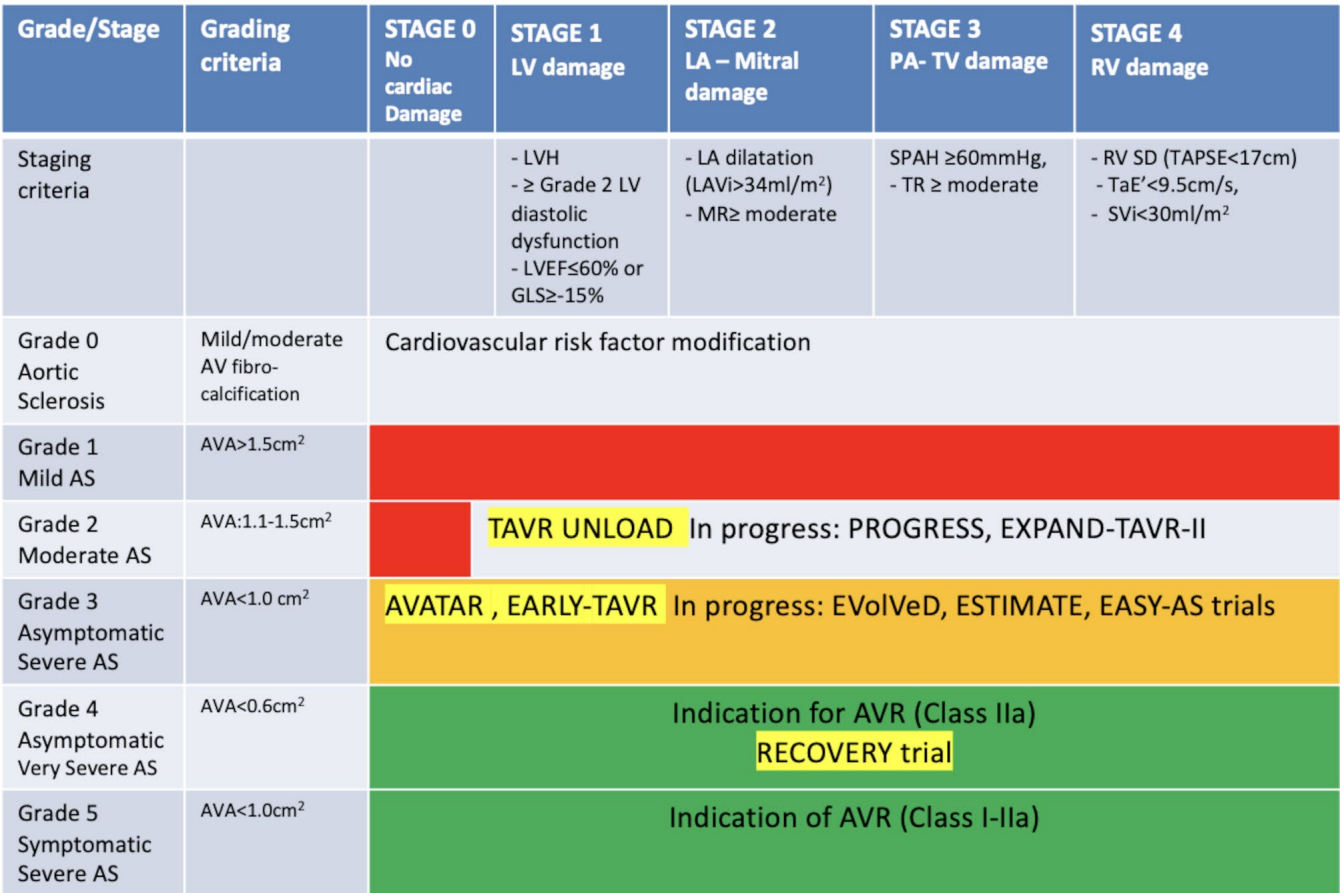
Proposal - Grading and Staging aortic valve stenosis in patients with preserved left ventricular ejection fraction PROGRESS- NCT04889872 at Clinical Trials.gov, TAVR UNLOAD: NCT02661451, EXPAND-TAVR-II: NCT05149755, AVATAR: NCT02436655, EARLY-TAVR: NCT03042104, EVolVeD: NCT03094143, ESTIMATE: NCT02627391, EASY-AS: NCT04204915, RECOVERY: NCT01161732 AVR: aortic valve replacement AVA: aortic valve area, LVH: left ventricular hypertrophy, LVEF: left ventricular ejection fraction, GLS: LV global longitudinal strain, LA: left atrium, LAVi: left atrial volume index, MR: mitral regurgitation, SPAH: systolic pulmonary pressure, TR: tricuspid regurgitation, RV: right ventricle, SD: systolic dysfunction, TaE’: Tricuspid annular E’ Doppler velocity, SVi: Stroke volume index, AS: aortic stenosis
